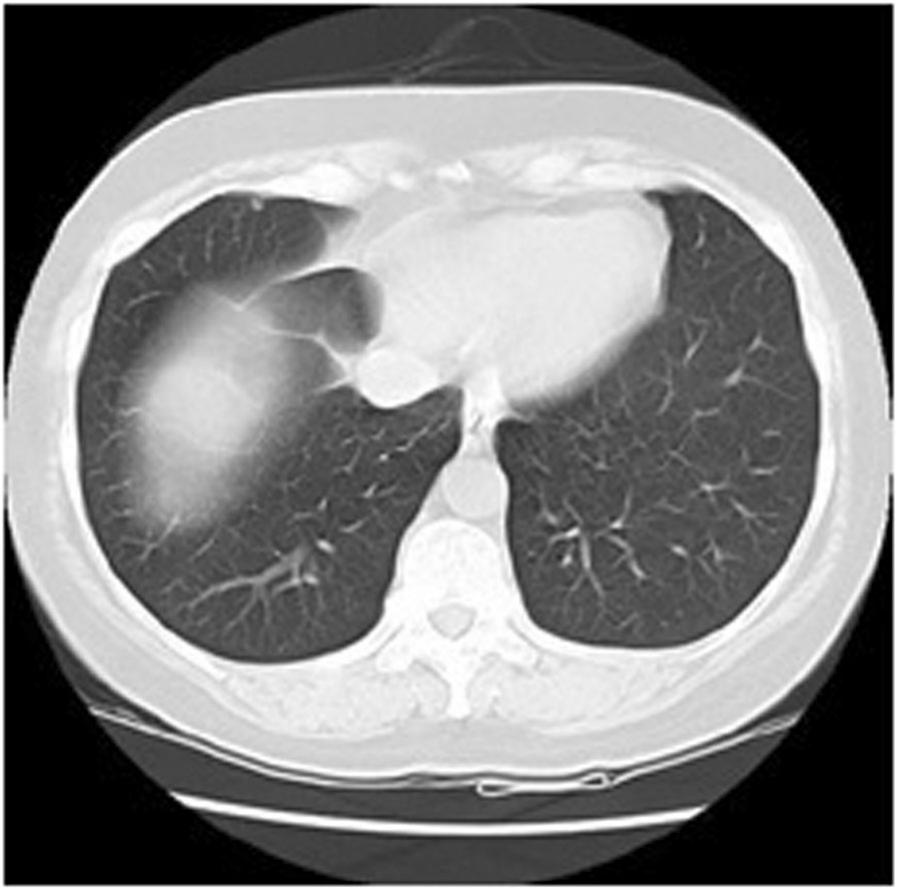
Original Lungs CT scan image.
